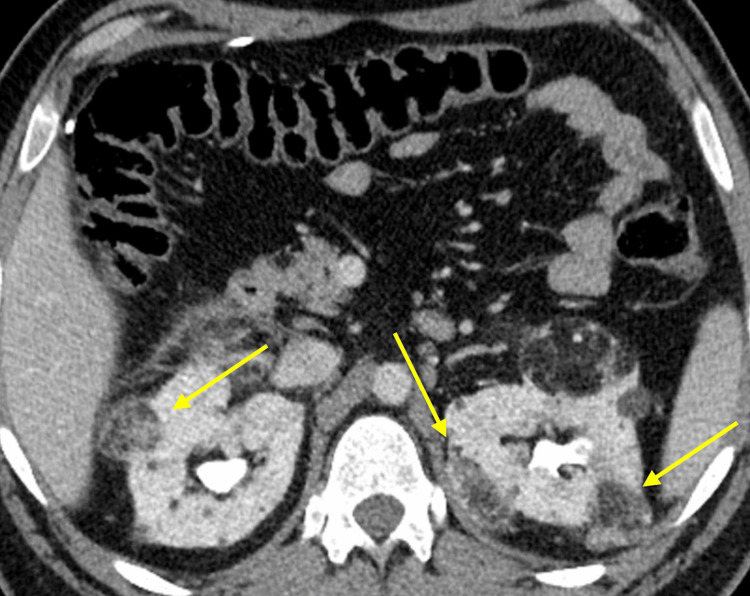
Bilateral renal angiomyolipomas on abdominal CT Axial CT images revealing multiple fat-containing hamartomatous lesions in both kidneys, indicated by yellow arrows.
